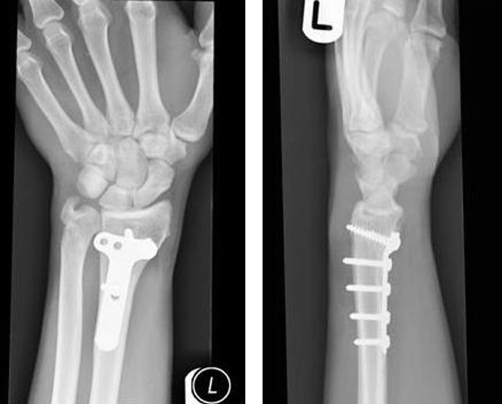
Follow-up radiographs of the same patient showing good radiological evidence of union of both radial and ulnar fractures.
